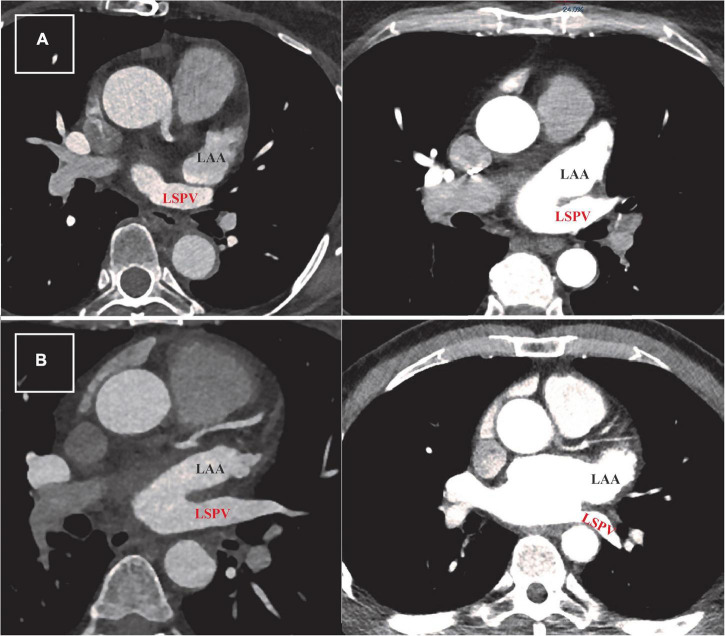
Defining abutting and non-abutting left atrial appendage–left superior pulmonary vein (LAA-LSPV). (A) Two examples of cardiac computed tomography (CT) scans from patients with abutting LAA (written in black) and LSPV (written in red). The measured distance between LAA and LSPV in these cases was less than 2 mm. (B) Two examples of CT scans from patients with persistent atrial fibrillation showing different trajectory of non-abutting LAA and LSPV. The measured distance between these two structures was more than 2 mm in both patients.
